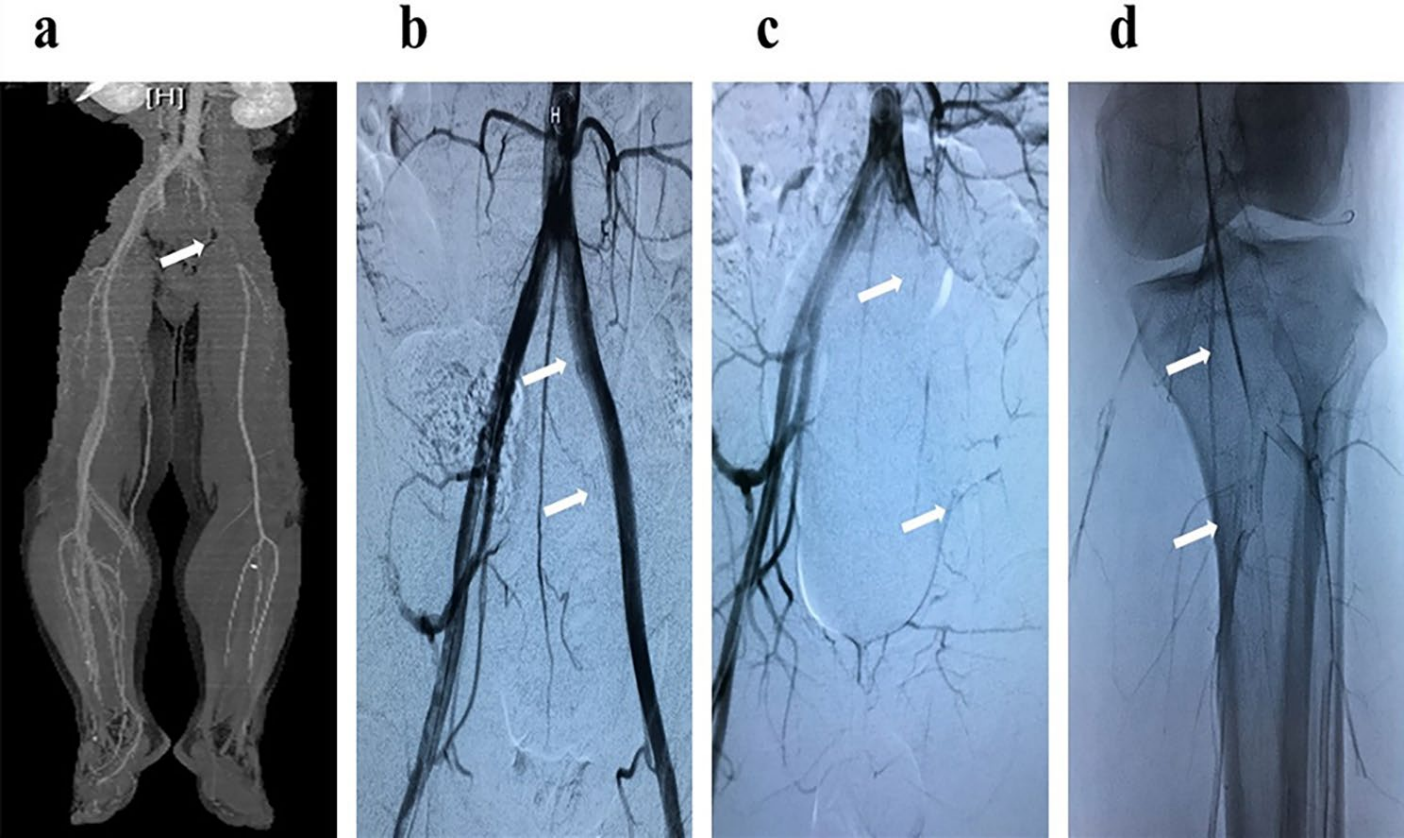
CTA showed (a) occlusion of the left iliac artery (arrow) and without grossly visible atherosclerotic lesions, arteriogram showed (b) complete recanalization (arrows) of the iliac artery after thrombectomy, and the second arteriogram showed complete occlusion (c) of the iliac and femoral vessels (arrows) and a filling defect (d) in the below-the-knee arteries (arrows)
